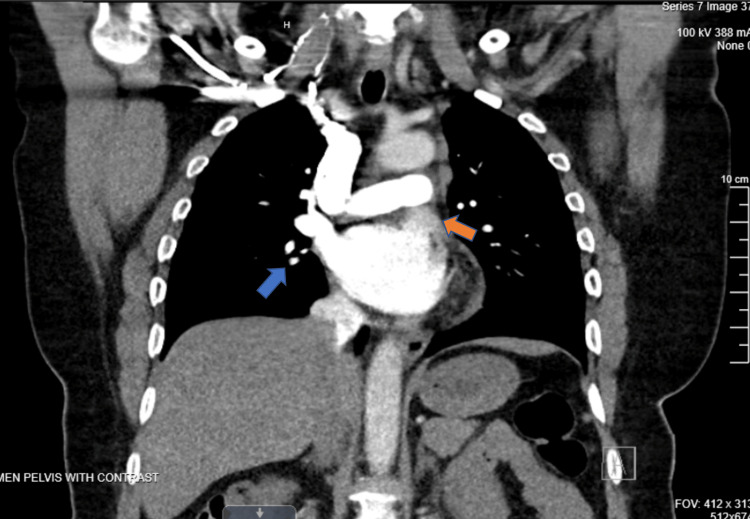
CT of the chest. Contrast-enhanced CT of the chest showing right hilar and mediastinal lymphadenopathy (orange arrow) and calcified subcarinal nodes (blue arrow). CT: computed tomography.
